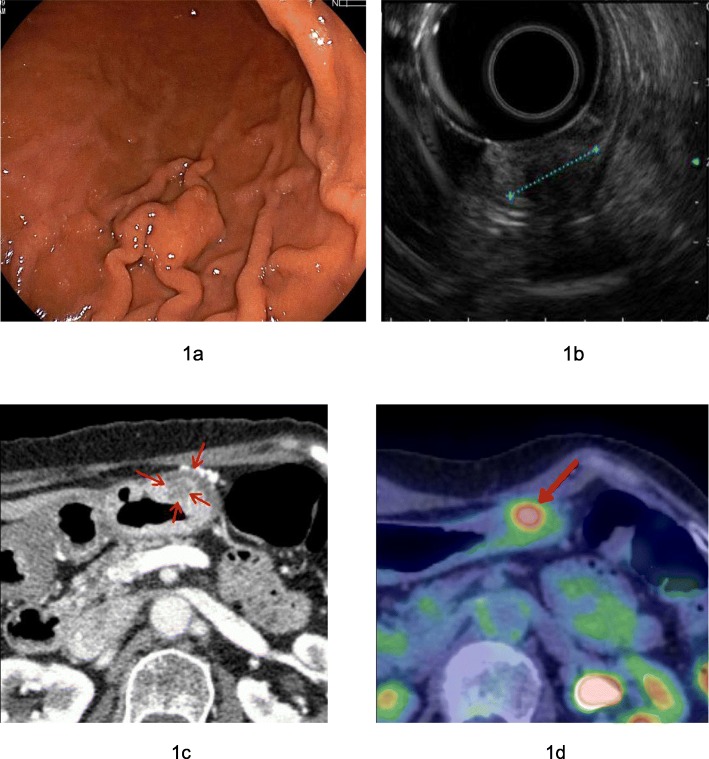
a Upper gastrointestinal endoscopy shows an elevated lesion in the greater curvature of the lower part of the stomach with no abnormalities of the gastric mucosa. b Endoscopic ultrasonography shows that the tumor is a low-echoic 10-mm lesion derived from the submucosal layer of the stomach. c Enhanced computed tomography shows a 15-mm hypovascular tumor in the greater curvature of the lower part of the stomach (arrows). d F-fluorodeoxyglucose (FDG) positron emission tomography shows an FDG uptake with an SUV_max_ of 3.8–5.1 (arrow)
